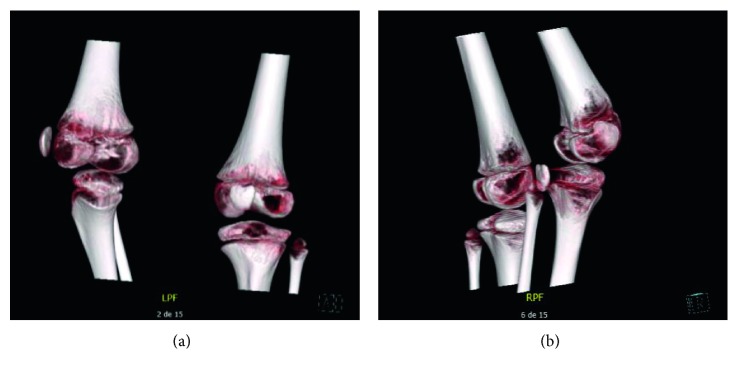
Patient 2: preoperative CT scan 3D reconstructions showing patella subluxation and femorotibial rotation (a) and normal state (b).
